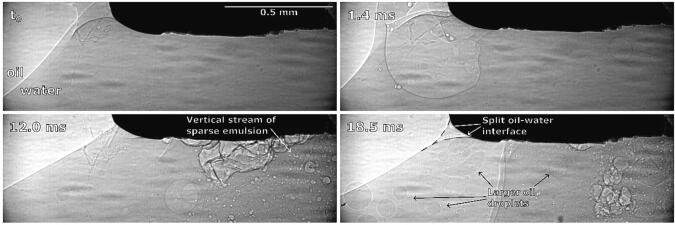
Synchrotron measurements of ultrasonic horn pushing parts of the oil phase into the water phase and subsequent emulsion formation.
